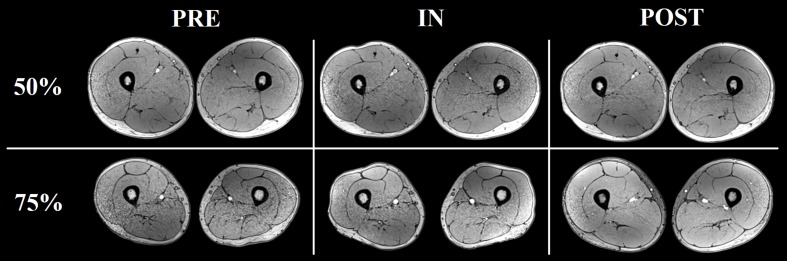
Representative increases in thigh muscles CSA (at ≈ 50% and ≈ 75% the length of the femur) as assessed by consecutive MRI scans before (PRE), and after 5 (IN) and 10 (POST) training sessions from one of the volunteers.
